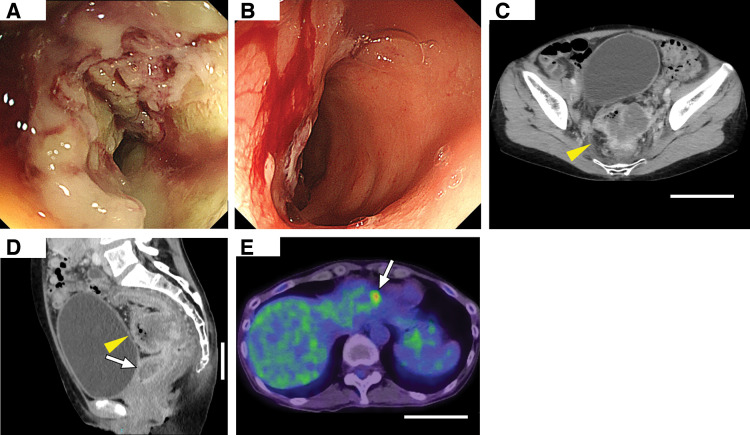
Endoscopic and radiologic findings at initial presentation (scale bar, 10 cm). (A) Lower gastrointestinal endoscopy revealed a type 1 tumor in the sigmoid colon. (B) Lower gastrointestinal endoscopy also demonstrated erosion in the rectum, suggesting possible invasion due to peritoneal dissemination. (C) Axial contrast-enhanced CT revealed the primary tumor in the sigmoid colon, measuring 62 × 44 mm. (D) Sagittal contrast-enhanced CT showed peritoneal dissemination in the Douglas pouch with suspected invasion into the rectum. (E) PET-CT showed a focal area of intense FDG uptake (SUVmax 5.7) in segment 3 (S3) of the liver, suggesting liver metastasis. FDG, fluorodeoxyglucose; SUVmax, standardized uptake value maximum
